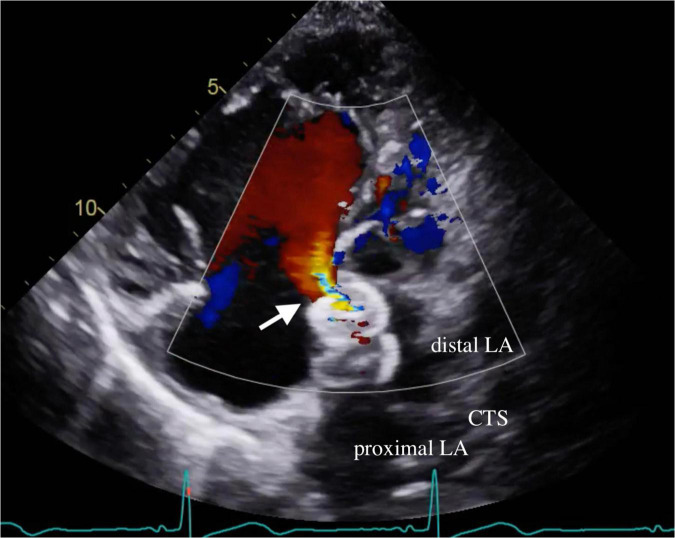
Residual ASD shunt. Transthoracic echocardiography demonstrated residual shunting (arrow) through the waist of the ASD device. ASD denotes atrial septal defect; CTS denotes cor triatriatum sinister; LA denotes left atrium.
